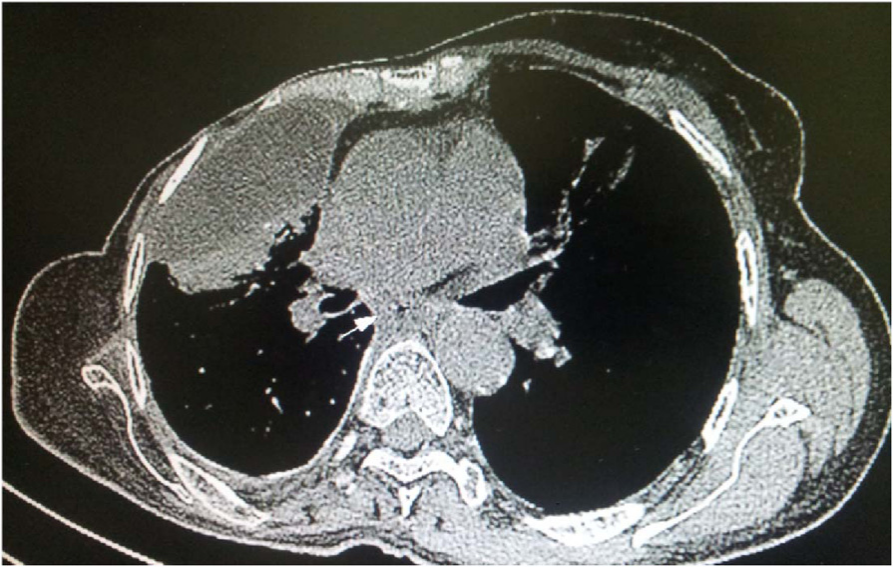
CT scan of circumferential thickening on thoracic esophagus.
